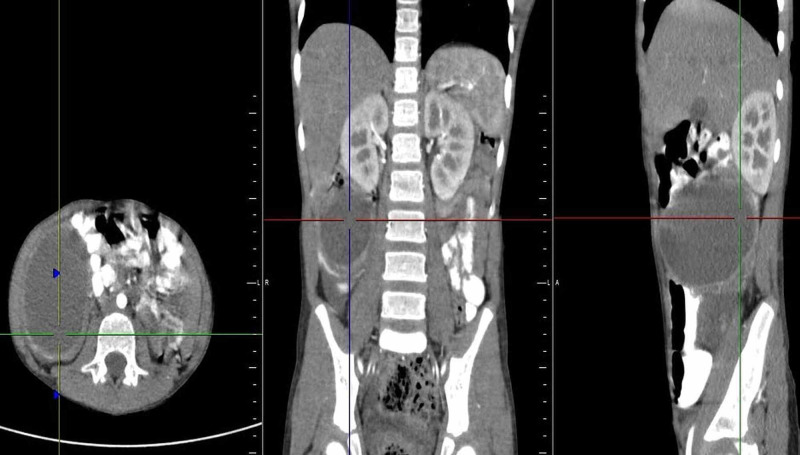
Contrast-enhanced CT image showing the size of the cyst and enhancement of the walls ( Center of the triangulation tool).
